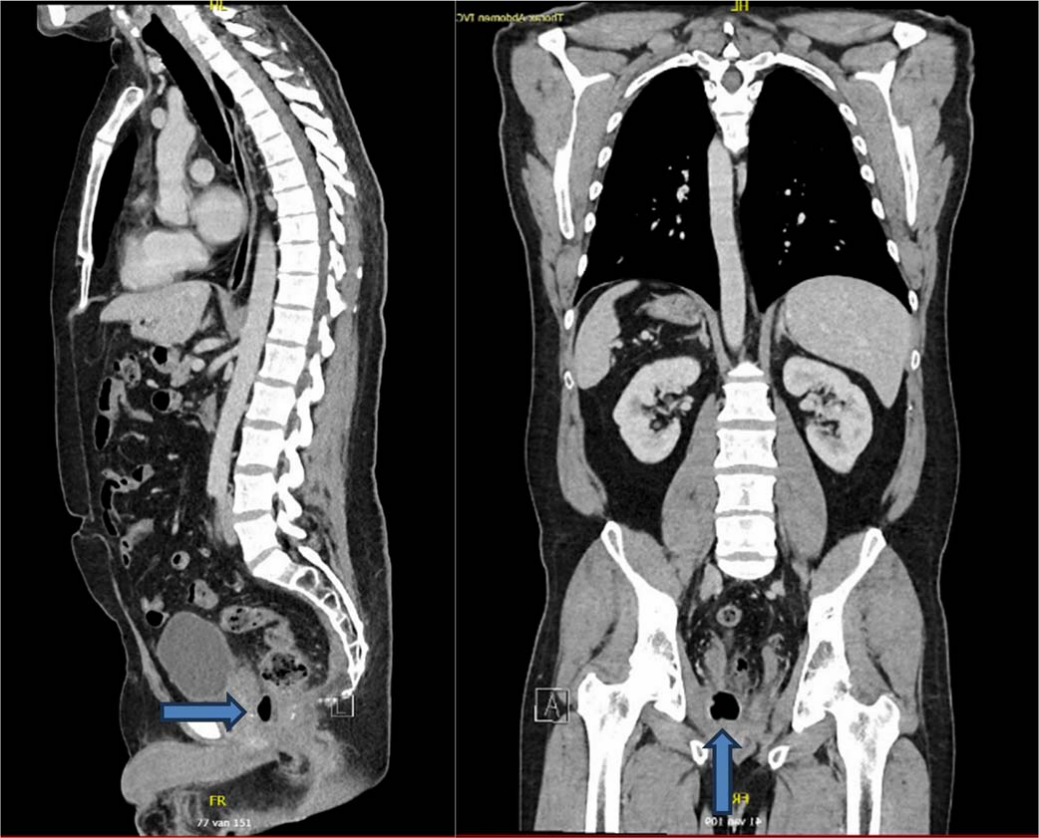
The CT scan of 1 week before the rectoscopy examination in case 1. The blue arrows indicate a cavity containing air and fluid measuring 3 cm in diameter, which connects to the fistula opening found during rectoscopy.
